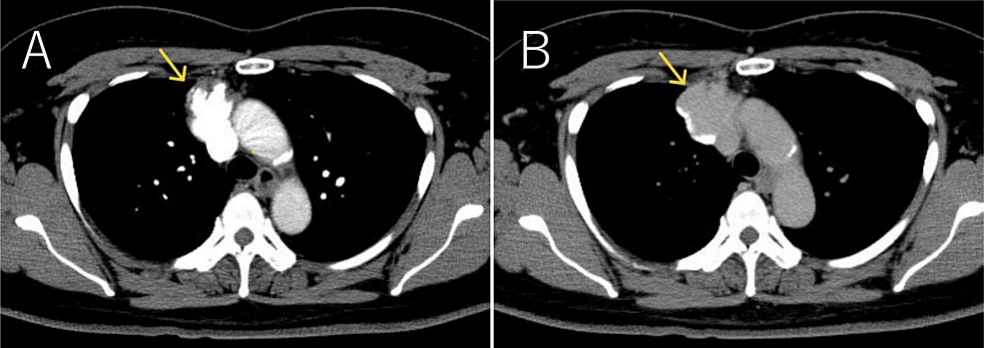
Dynamic contrast-enhanced CT (injected via the arm) Dynamic contrast-enhanced CT injected via the arm showed early and persistent enhancement of the mass, with contrast material pooling on the dorsal side in the delayed phase.
